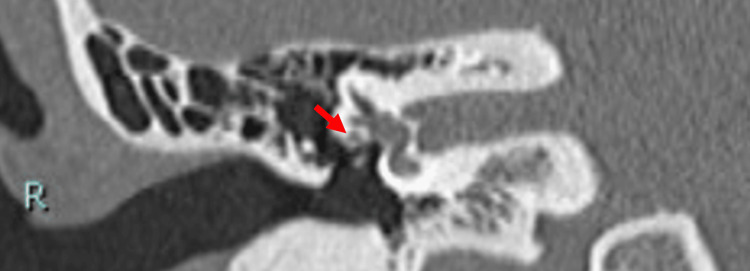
Coronal HRCT images of the right temporal bone showing the normal position of facial nerve (arrow) below the lateral semicircular canal (Case 1). HRCT, High-resolution computed tomography
